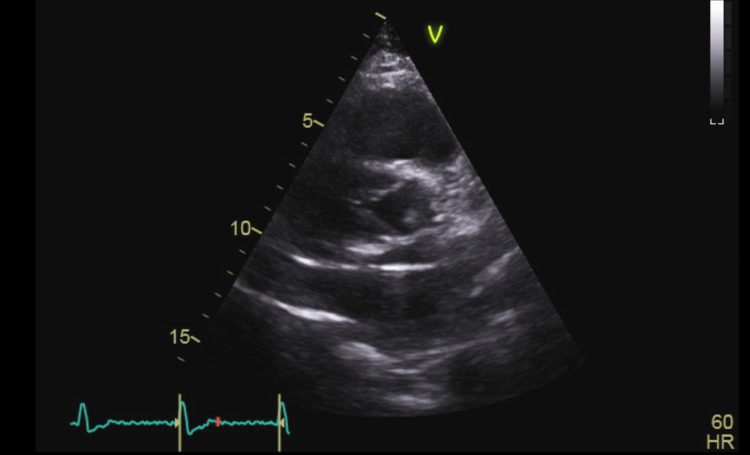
PSAX TTE view at the level of aortic valve with the lead passing through the PFO from the RA to the LA PSAX: Parasternal short axis; TTE: transthoracic echocardiogram; PFO: patent foramen ovale; RA: right atrium; LA: left atrium
